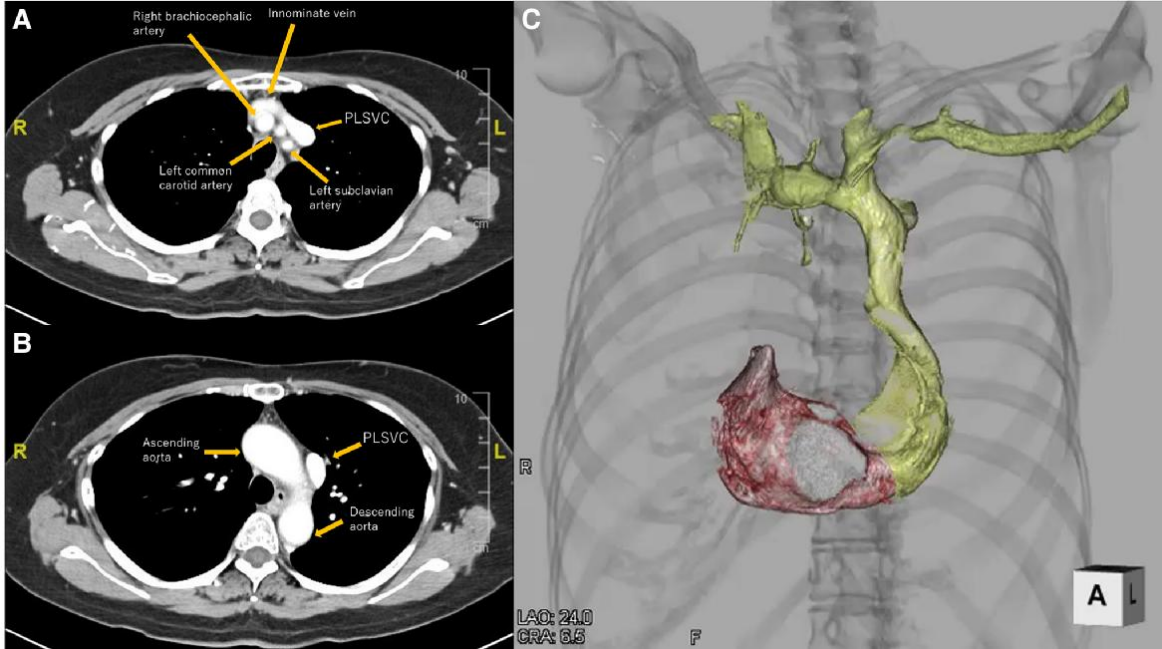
Cardiac computed tomography. (*A*) Axial view at the level of the superior margin of the sternum. (*B*) Axial view at the level of the sternal angle. (*C*) Reconstructed 3D image of the subclavian vein, persistent left superior vena cava, right atrium, and right ventricle. PLSVC, persistent left superior vena cava.
